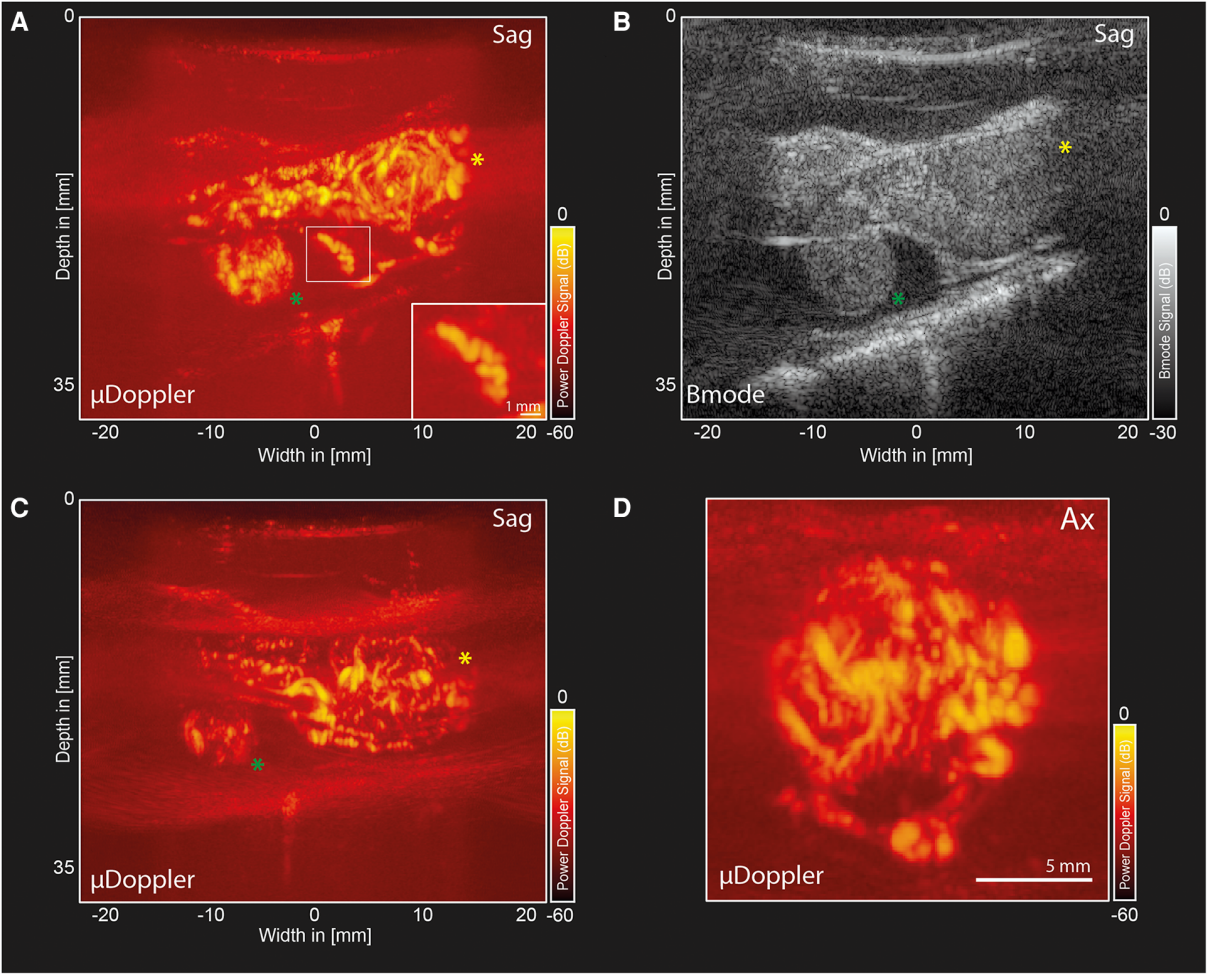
µDoppler-images of two hemangioblastoma foci. (A) Sagittal µDoppler-image spanning both hemangioblastoma foci. Lesion 1 (*yellow asterix*) and Lesion 2 (*green asterix*). The zoom-in panel shows an interesting vascular detail on what seems to be the contour of the healthy myelum. (B) Bmode corresponding to the µDoppler-image in panel A. (C) Sagittal µDoppler-image, again spanning both hemangioblastoma foci. (D) Axial image of the most cranial (*yellow asterix)* hemangioblastoma. Sag, Sagittal; Ax, Axial.
